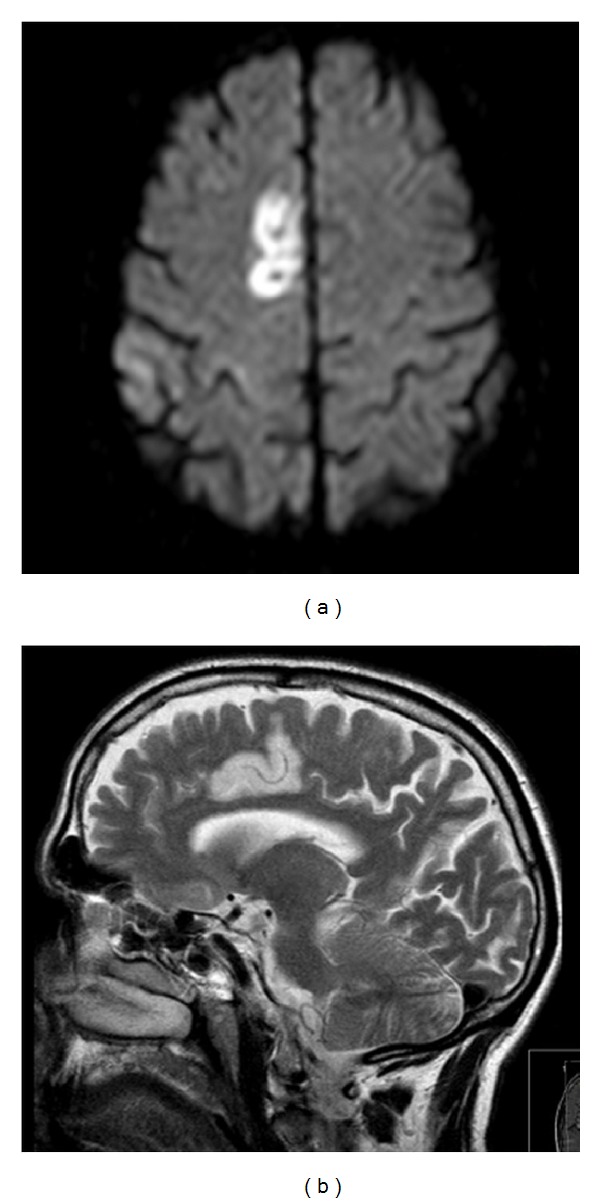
(a) Diffusion-weighted image and (b) T2-weighted image: acute ischaemic lesion on the inner surface of the frontal lobe, extending to the right cingulate gyrus.
